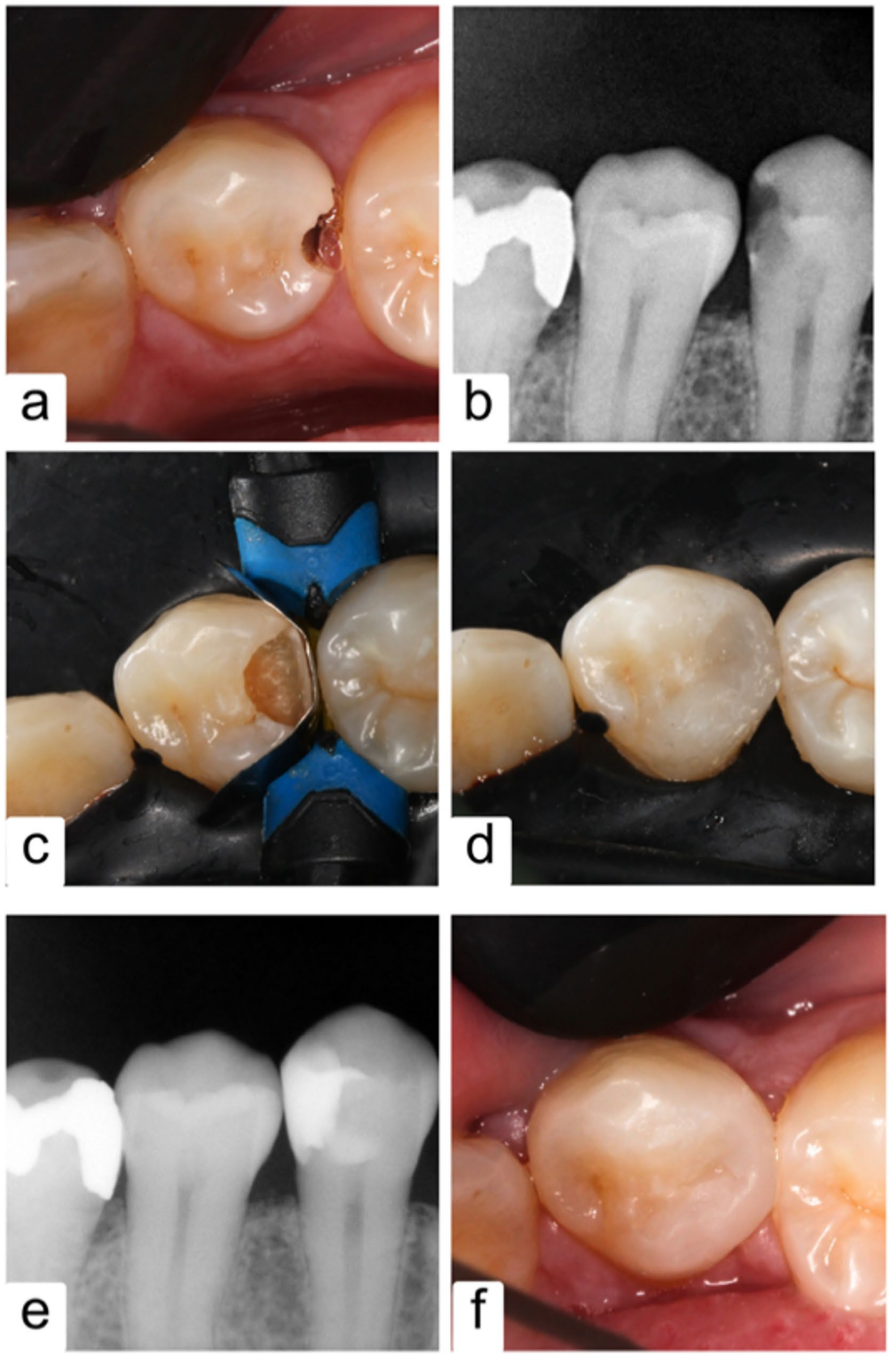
Photographs of the clinical procedures of a restoration; a: Preoperative clinical picture; b: Preoperative X-ray; c: Rubber dam isolation and matricing application; d: XF restoration immediately postoperative; e: Postoperative X-ray; f: Restoration after finishing and polishing
